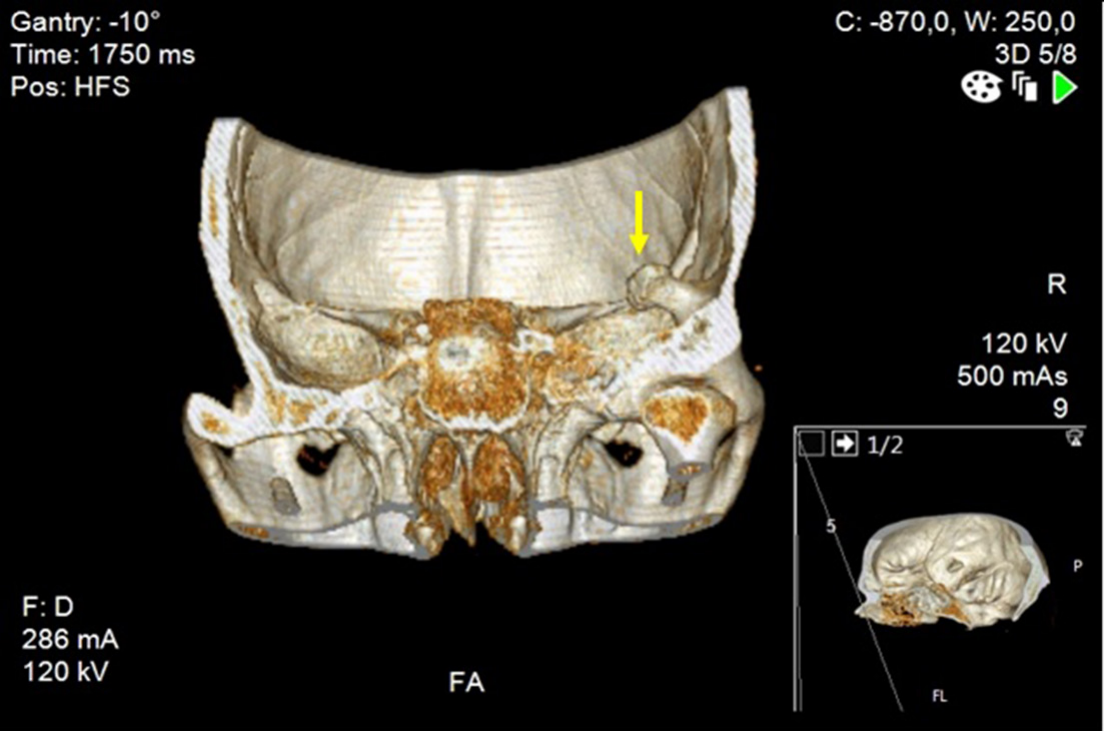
3D reconstruction-CT of the enostosic lesion (yellow arrow)
